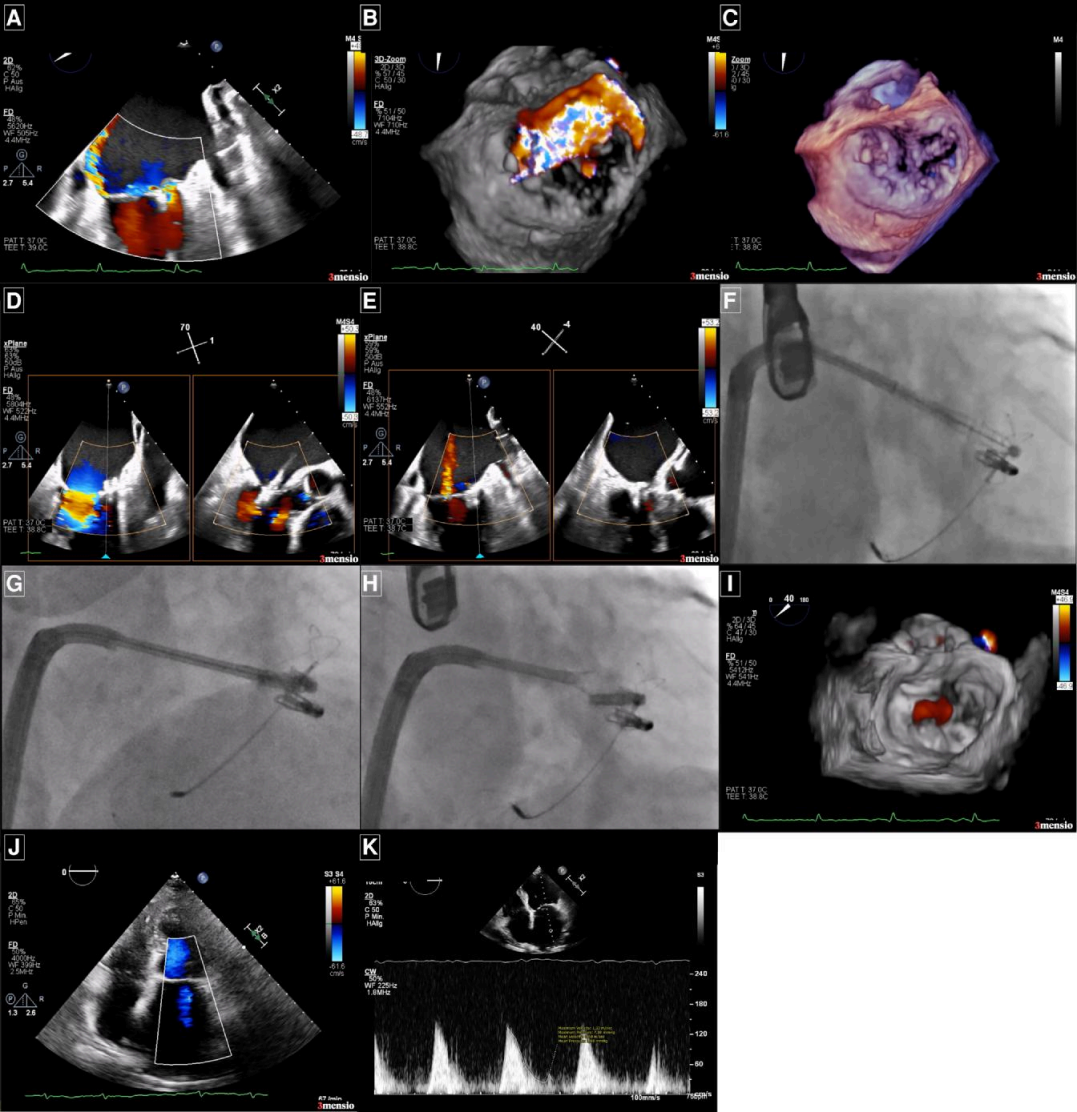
Diagnostic and intraprocedural echocardiographic and fluoroscopic imaging of the mitral valve before and after the second mitral transcatheter edge-to-edge repair procedure. (*A*) Mitral commissural view with colour Doppler showing the previously implanted clip at the A1/P1 segment, with a recurrent eccentric regurgitant jet originating from the lateral commissure. (*B*) 3D en face view of the mitral valve during systole, with the aortic valve at 12 o'clock, showing the eccentric jet arising lateral to the initial clip in the A1/P1 segment. (*C*) 3D transoesophageal echocardiography image revealing the residual prolapse of the posterior mitral valve leaflet responsible for the severe recurrent mitral regurgitation prior to the second mitral transcatheter edge-to-edge repair procedure. (*D*) Intraprocedural biplane imaging during device positioning with the PASCAL device (Edwards®), targeting the area between the first clip and the lateral commissure. (*E*) Demonstration of significant reduction in mitral regurgitation after deployment of the second device. (*F*, *G*, *H*) Fluoroscopic images capturing catheter approach to the target landing zone. (*I*) 3D view en face after second clip deployment. (*J*) Post-procedural transthoracic echocardiography showing only trace residual mitral regurgitation. (*K*) A mean transmitral gradient of 2.0 mmHg was recorded following the second device implantation.
